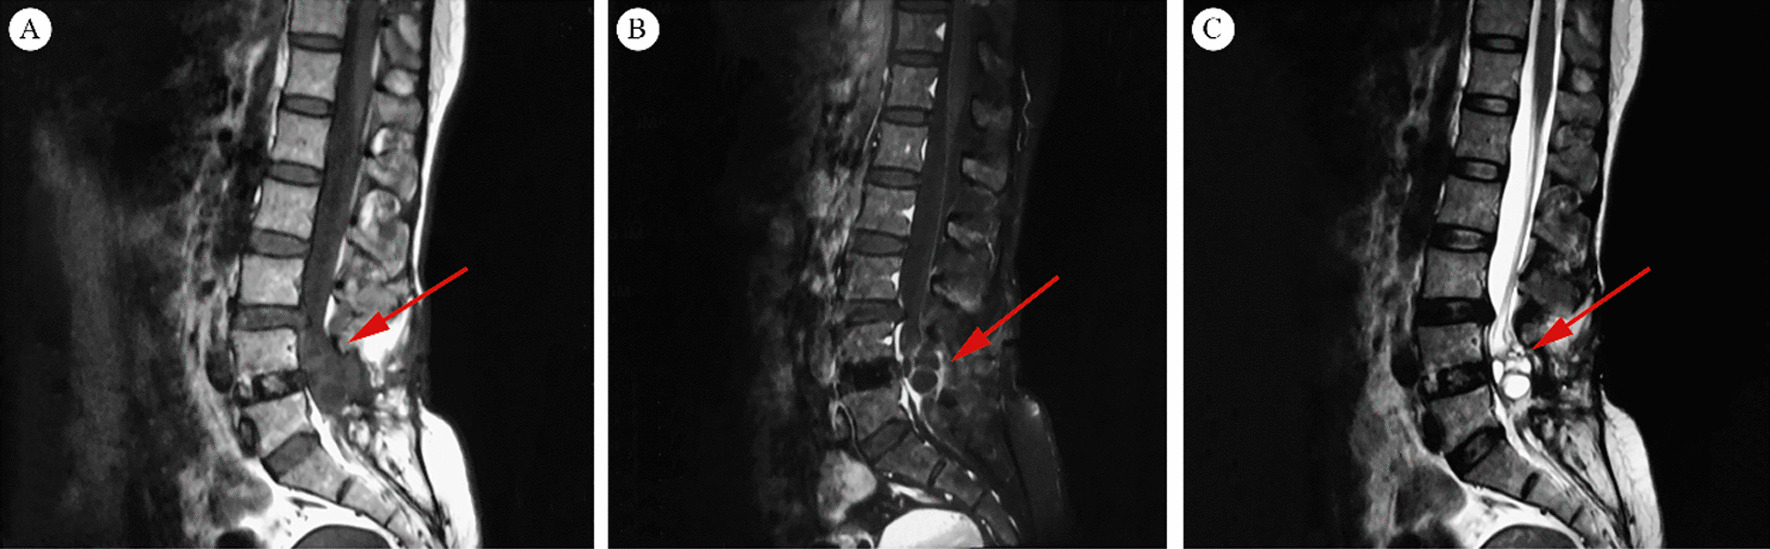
Magnetic resonance images of the lumbar spine. A Sagittal T1-weighted magnetic resonance imaging showing multiple low signal intensity lesions (red arrow). B Sagittal T1-weighted magnetic resonance imaging with intravenous gadolinium contrast medium showing the same lesion with low signal intensity, but the edge is enhanced (red arrow). C Sagittal T2-weighted MRI showing multiple high signal lesions (red arrow)
